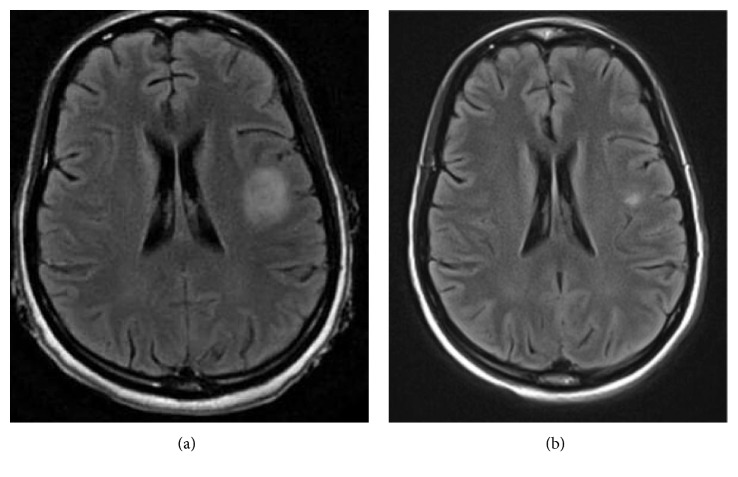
(a) MRI brain with and without contrast on admission (T2-FLAIR image) demonstrating focal mass in the dorsal left insular cortex and subcortical white matter. There is surrounding edema with little mass effect. (b) MRI brain at a 10-day interval follow-up demonstrating marked improvement in the dorsal left insular brain lesion (T2-FLAIR image).
